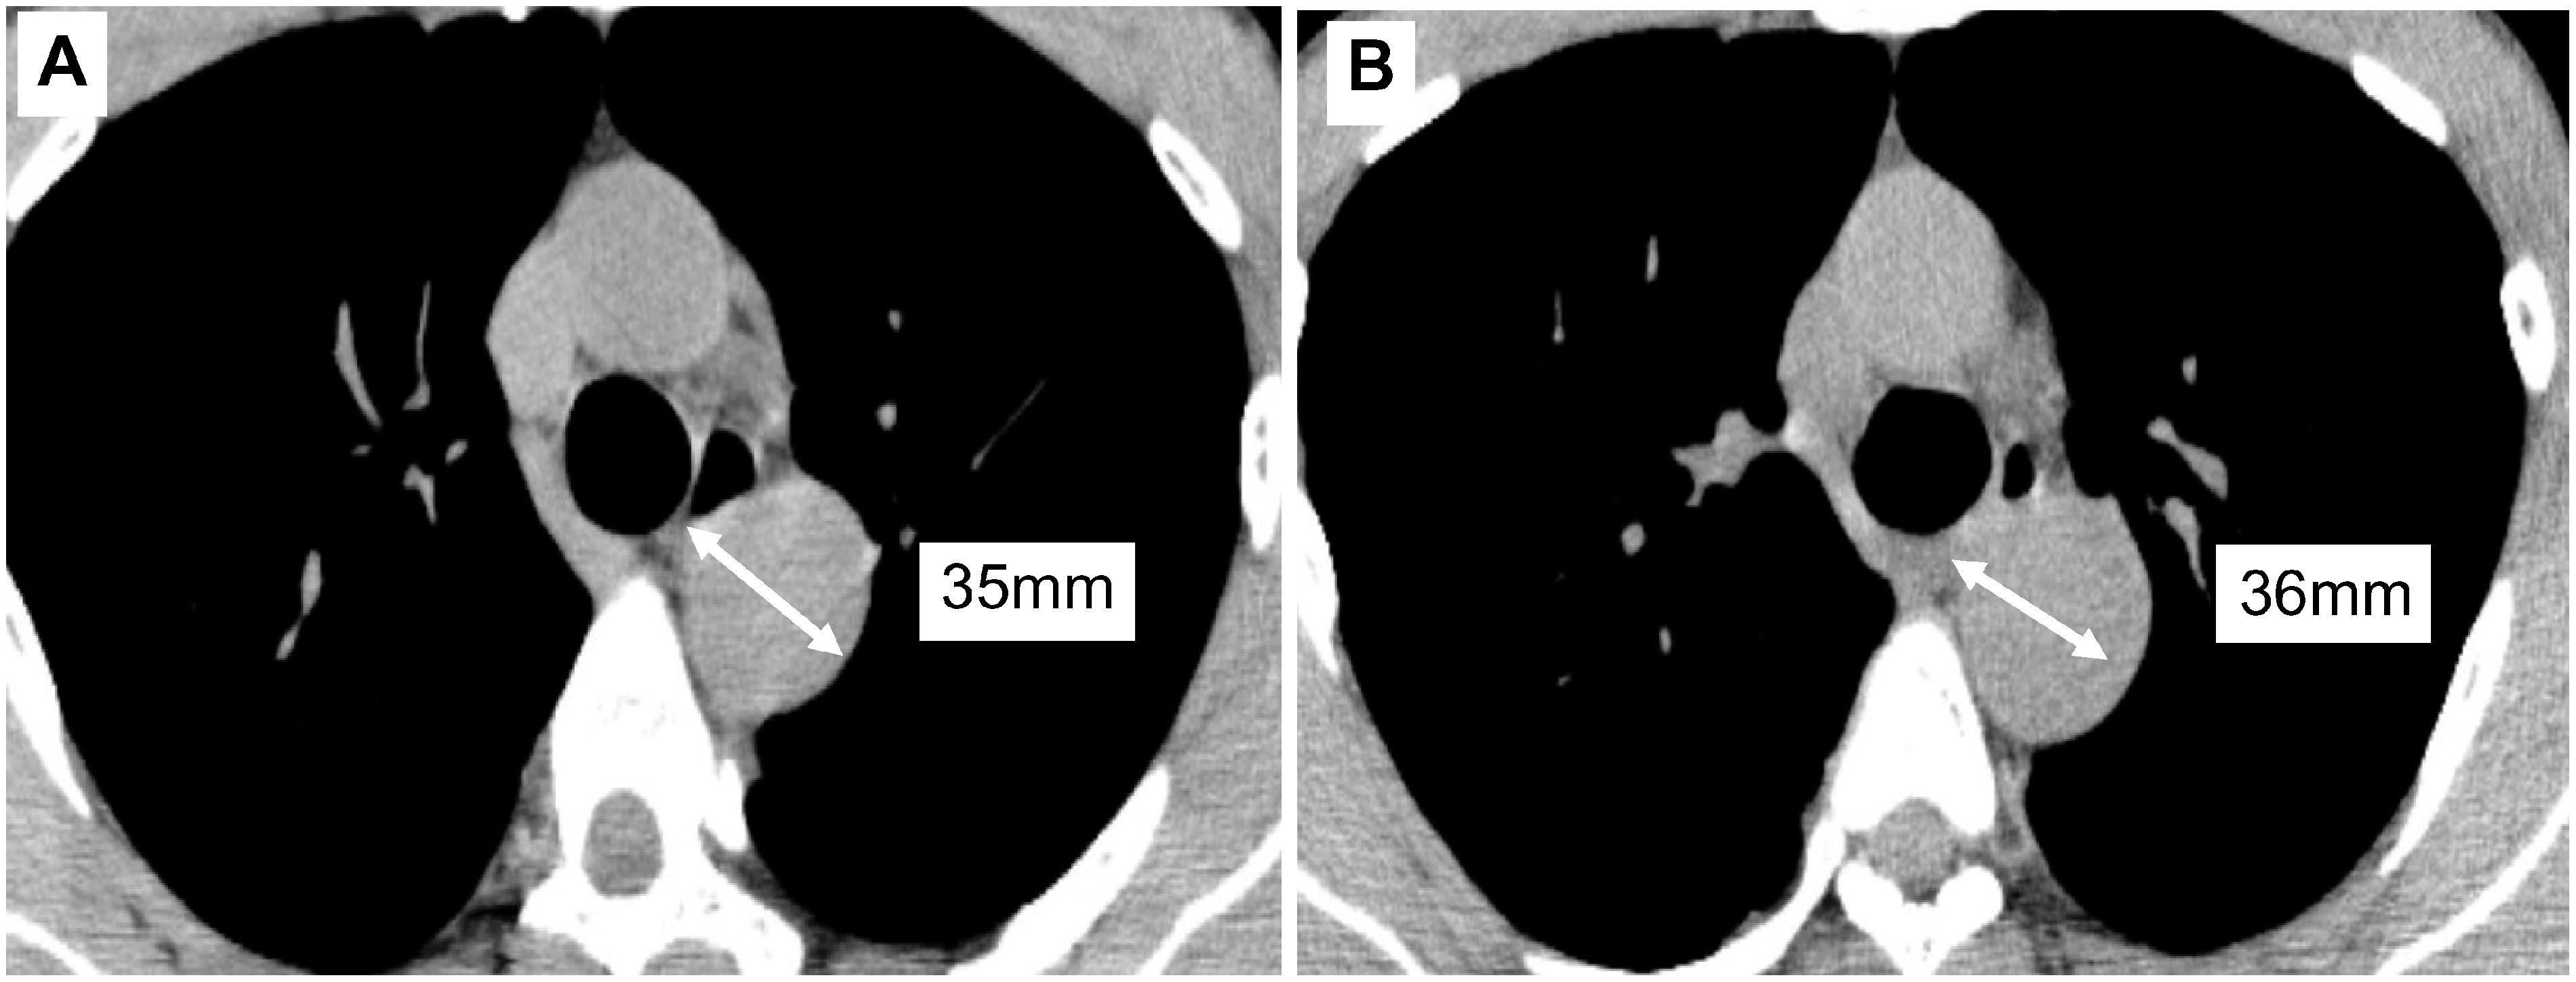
Fig. 2 (A) Computed tomography (CT) at 3 months after discharge. Maximum axial diameter of the descending aorta, 35 mm. (B) CT performed at 9 months after discharge showing the maximum axial diameter of the descending aorta as 36 mm.
